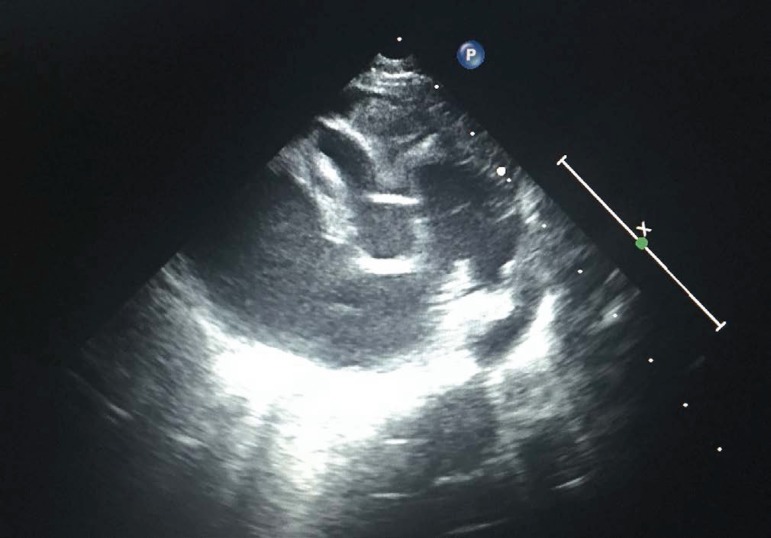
A transthoracic image of CAVF from the right coronary artery to right
